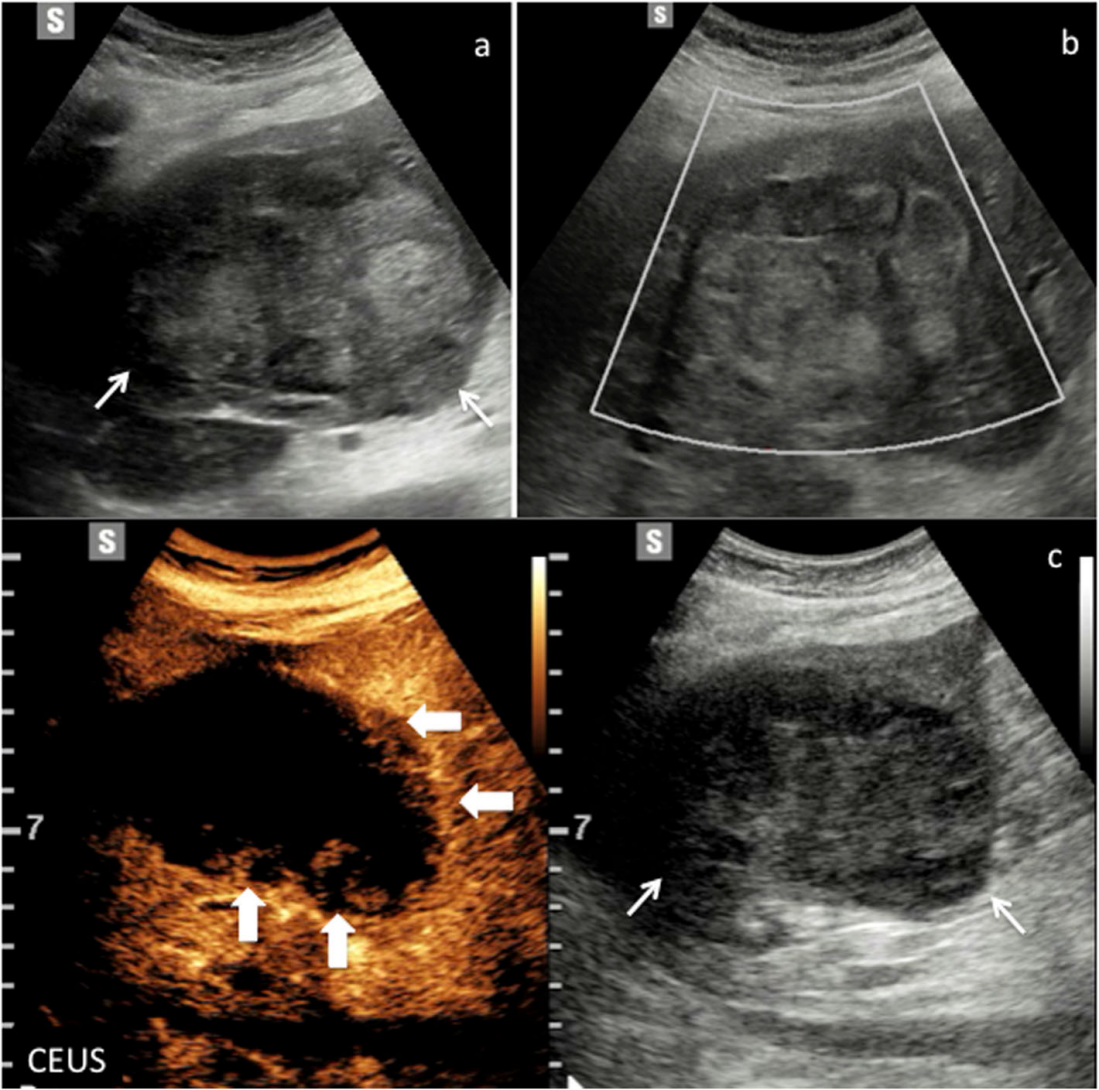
CEUS LI-RADS M (probably or definitely malignant but not HCC specific) nodule. Cholangiocarcinoma in a 87-year-old man with virus B-related cirrhosis. Baseline US image shows a markedly heterogeneous lesion sized 8.5 cm in the segment VI (a) (arrows). No vascular signal is detectable at color-Doppler (b). At CEUS (c), the nodule shows rim enhancement surrounding a constantly avascular area (arrows)
